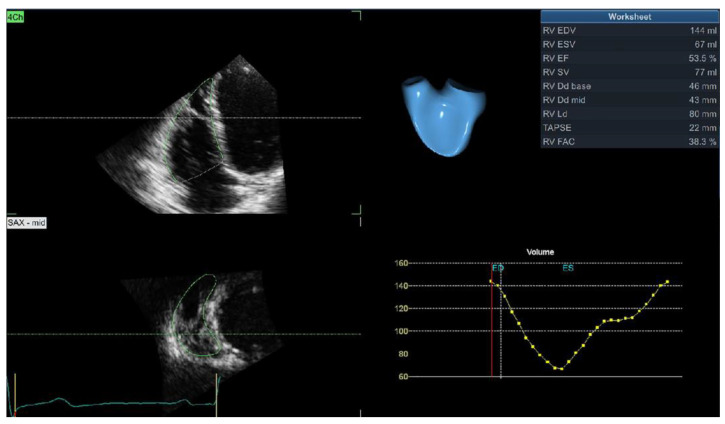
3D echocardiographic evaluation of right ventricular volume and ejection fraction.
